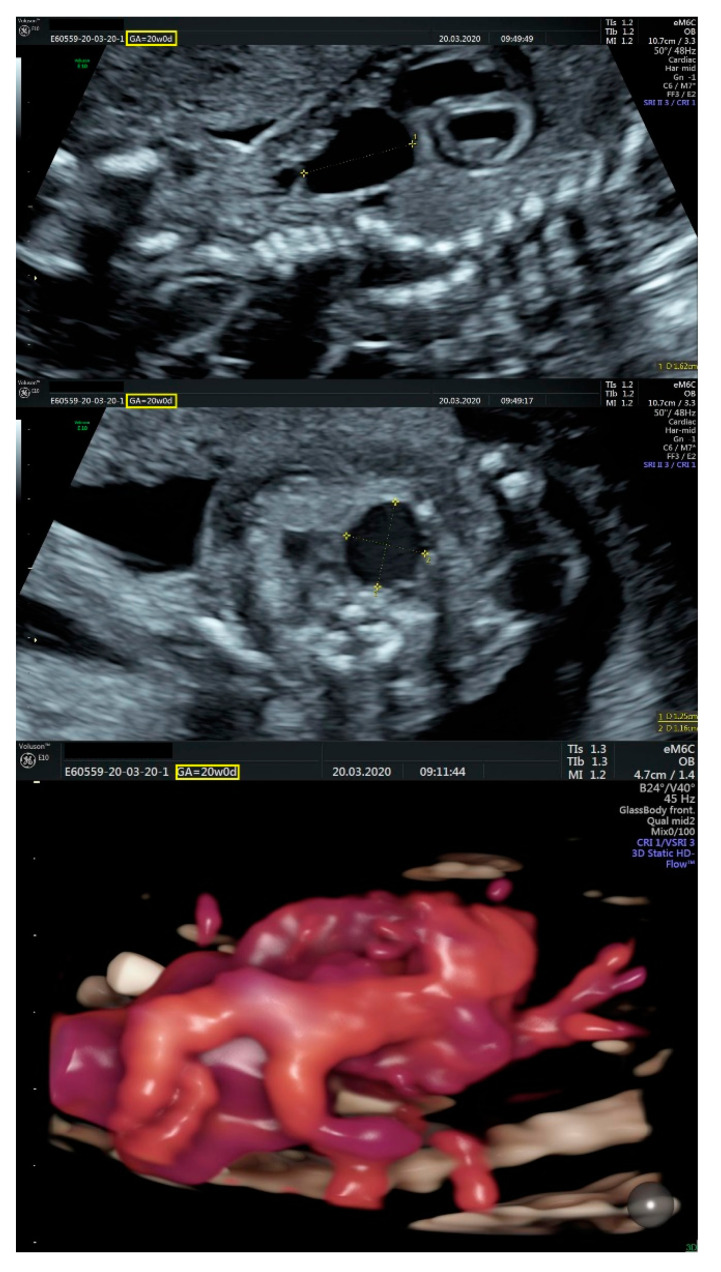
(Top and middle): ultrasonographic measurements of the aneurysm in sagittal (top) and transversal (middle) incidences; (bottom): 3D reconstruction of the mediastinal vessels.
